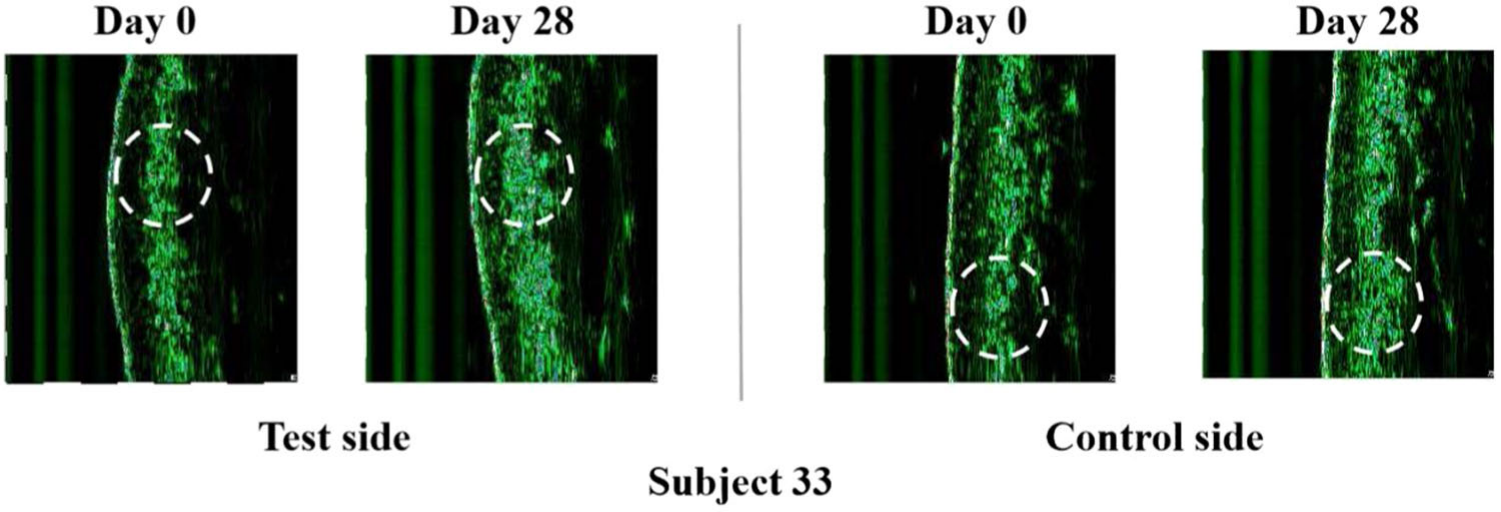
Images of skin condition at baseline and on D28. Ultrasonographic skin aspect on the test and control sides in Subject 33, the white circle represents the dermis.
